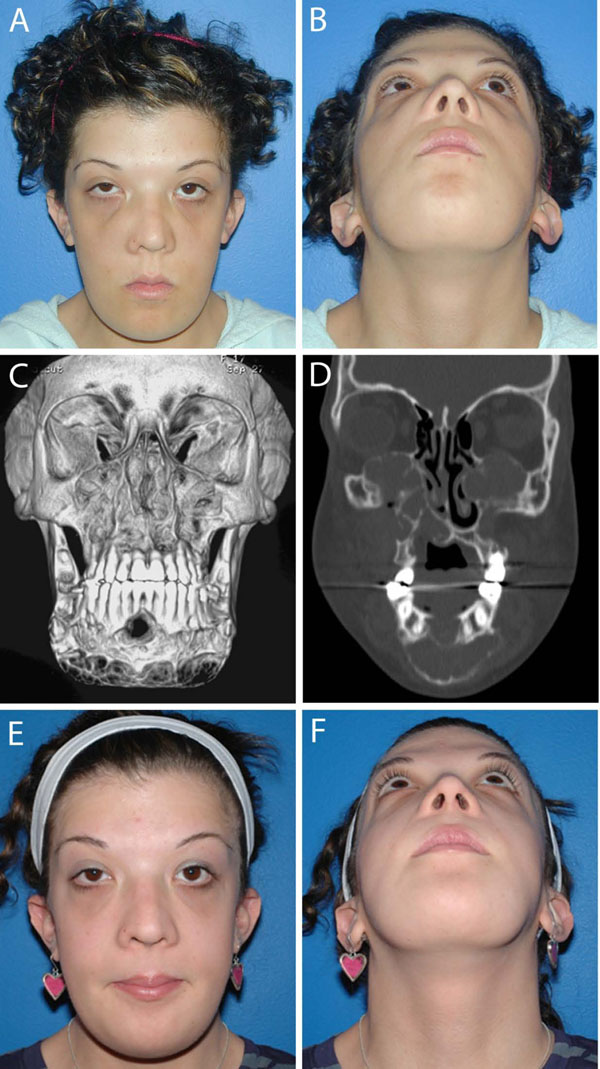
Noonan/multiple giant cell lesion syndrome. A 20-year old woman with Noonan/multiple giant cell lesion syndrome with bilateral involvement of the mandible and the maxilla. A and B. Frontal and submental photographs reveal prominent maxillary and mandibular contours, slight frontal bossing and increased intercanthal distance, downslanting palpebral features, epicanthal folds and posteriorly angulated ears. Lesions were painless to palpation. C and D. Coronal and 3D CT scan at initial presentation demonstrate involvement of the maxilla, including the maxillary sinuses and the nasal-maxillary region and the mandible from the antegonial notch to antegonial notch. The lesions are expansile and mixed radiolucent - radiopaque with cortical thinning and expansion into the orbits bilaterally. Patient was positive for a Noonan syndrome mutation in *SOS-1*. She underwent a contour resection of the maxillary and the mandibular giant cell lesions in two sessions. Postoperatively, she was treated with 4 courses of zoledronic acid, given at 6-week-intervals. E and F. Post-treatment frontal and submental photographs.
